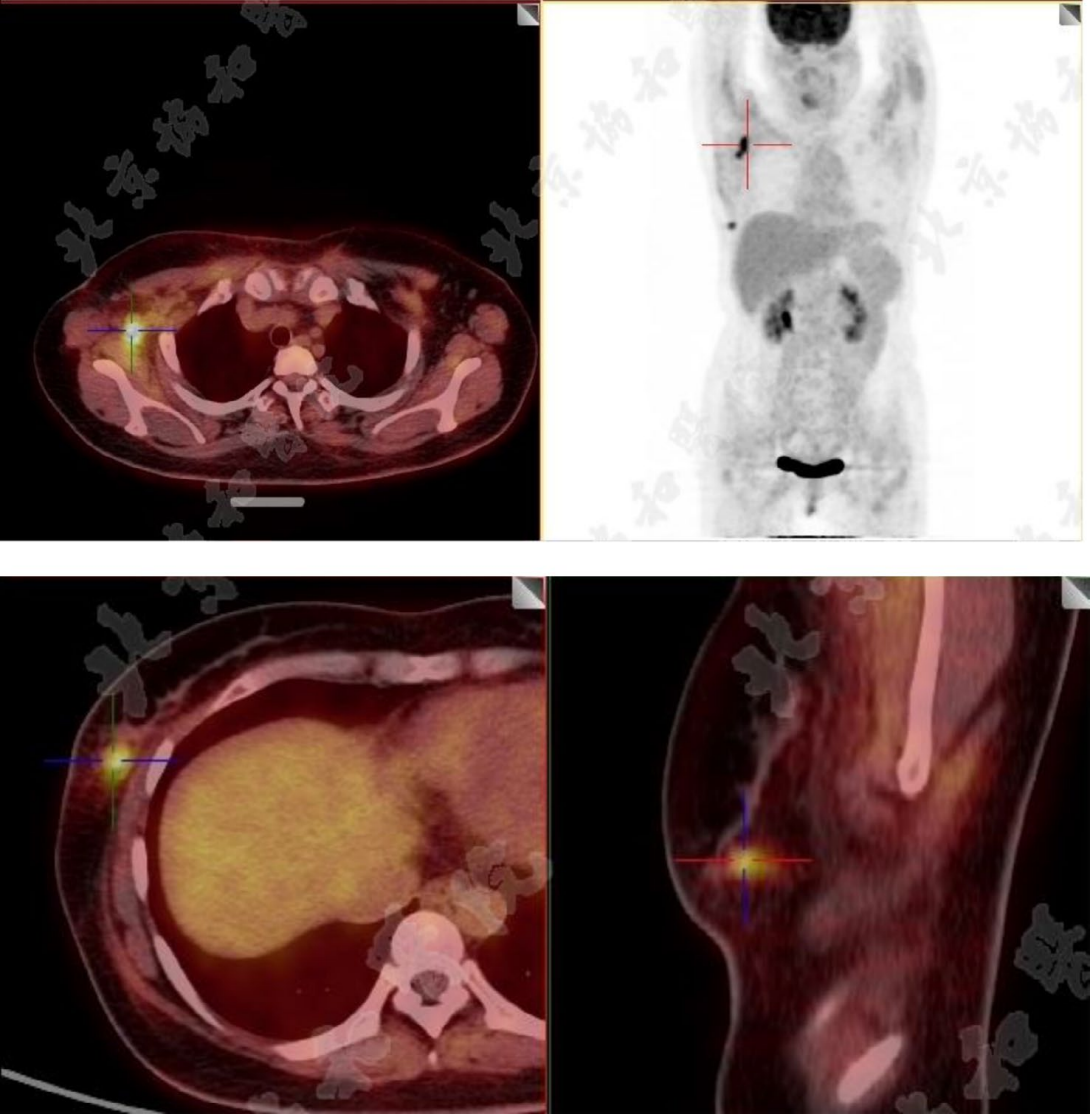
PET‐CT showed right‐sided breast cancer and multiple lymph node metastases in the armpit.
